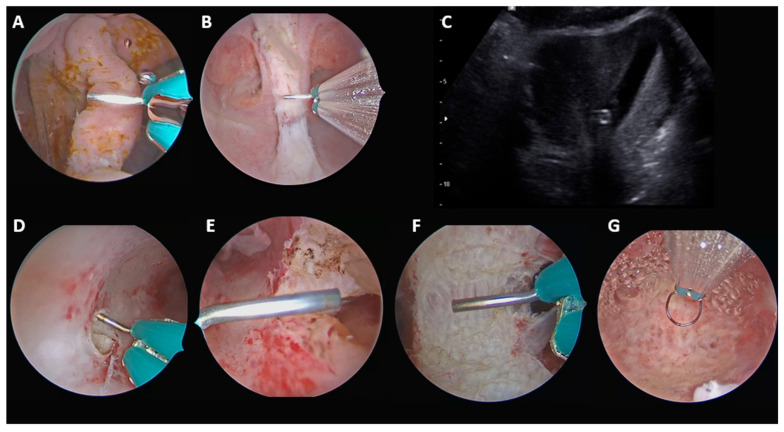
U2bC2V1, without communication between the two hemiuteri. (A) Beginning of vaginal septum incision. (B) Completion of vaginal septum incision up to the level of the two cervices. (C) Real-time ultrasound view guiding the supracervical incision. (D) Supracervical incision to create communication between the two uterine hemicavities under transabdominal ultrasound guidance. (E) Creation of communication between the two uterine hemicavities, allowing subsequent uterine septum incision. (F) Incision of the uterine septum. (G) Final panoramic view of the uterine cavity.
